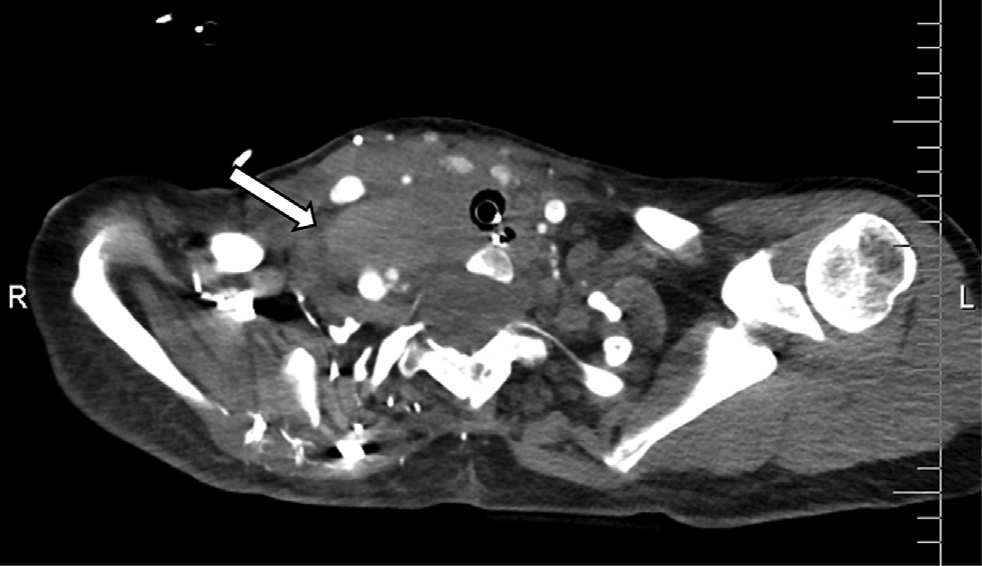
CT soft tissue neck with contrast demonstrating soft tissue prominence in the right supraclavicular region with a blush of contrast posteriorly suggestive of evolving hematoma with arterial extravasation.
